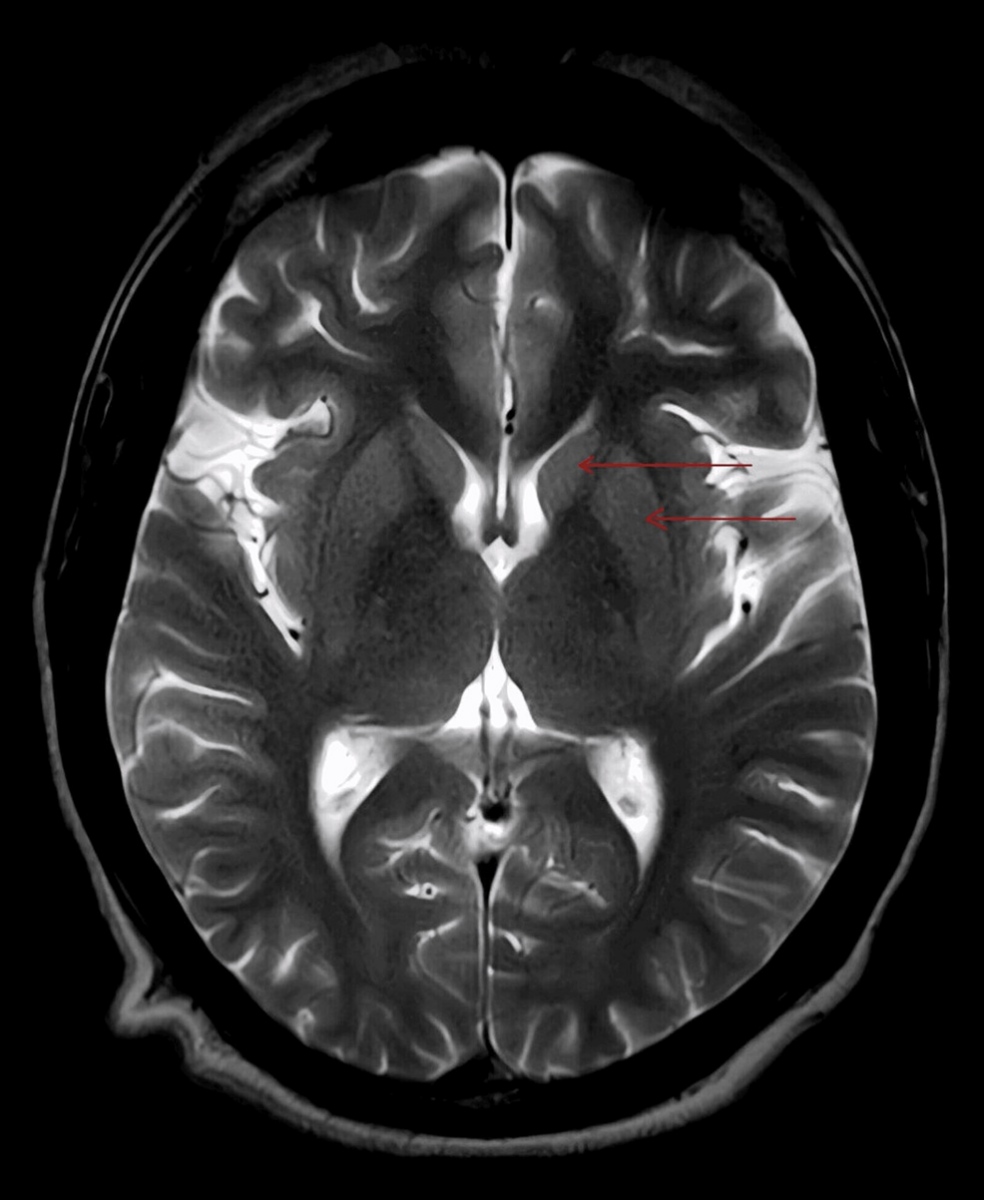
This shows an MRI T2-weighted image with no obvious abnormality in the basal ganglia (red arrows showing basal ganglia)
